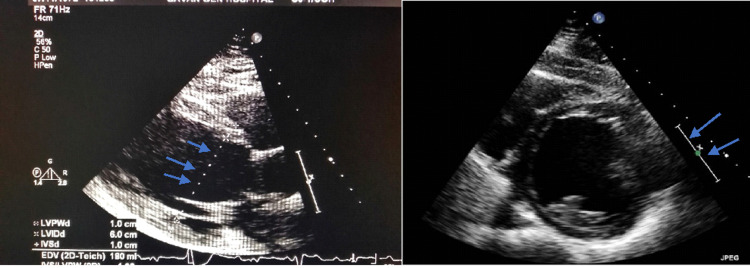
Echocardiogram showing a dilated left ventricle (arrows point to the LV dimension of 6.0 cm) with global hypokinesia. LV: Left ventricular
